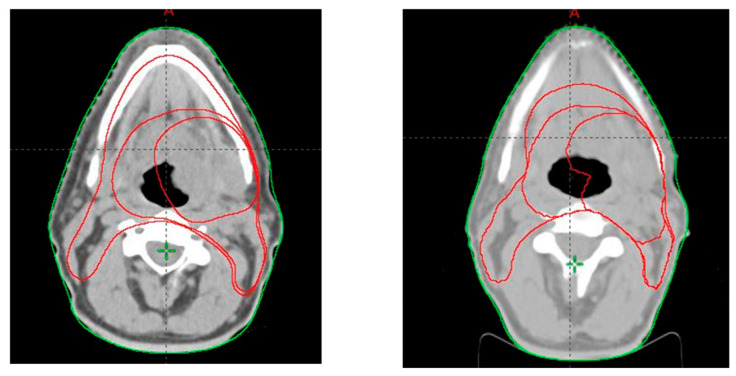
Tumor volumes contoured at first CT scan (left) and second CT scan after completion of 18 radiotherapy fractions (right).
